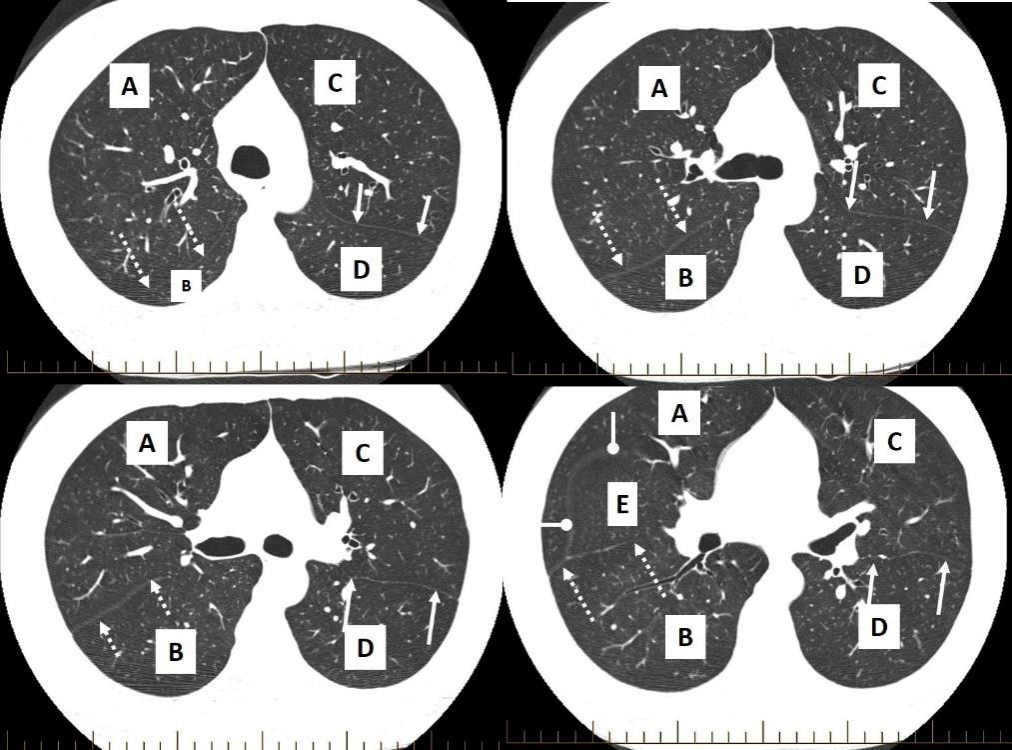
Multiple axial CT images (lung window) of the chest of a 51 years old man are shown. Identify the structures depicted as white lines and marked by dotted, solid and oval arrows in both the lungs. Also name the lobes in both the lungs labelled A to E
